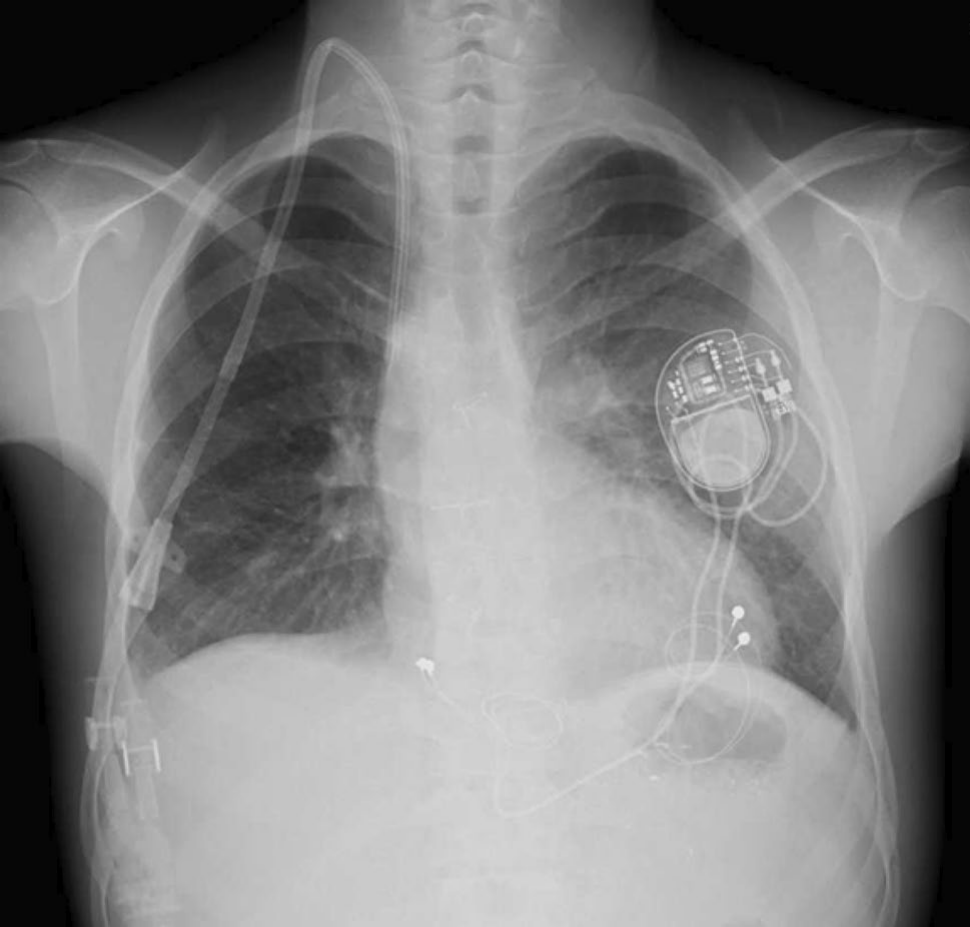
Chest X-ray after insertion of a tunneled hemodialysis catheter. The tip of the tunneled hemodialysis catheter was presumed to be located in the extracardiac Fontan conduit. A permanent pacemaker was implanted at the same time
